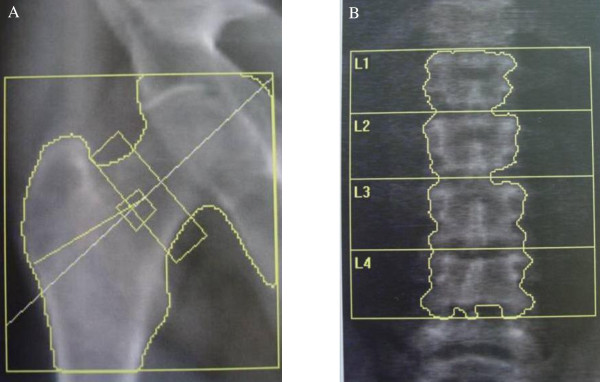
Dual-energy X-ray absorptiometry (DEXA) assessment of bone mineral density of the femoral neck (A) and the lumbar spine (B): T scores of - 4.2 and - 4.3 were found at the hip (A) and lumbar spine (B), respectively in a 53 year-old male patient affected with Fabry disease. Courtesy: Dr Caroline LEBRETON, CHU Raymond Poincaré, Garches, France.
